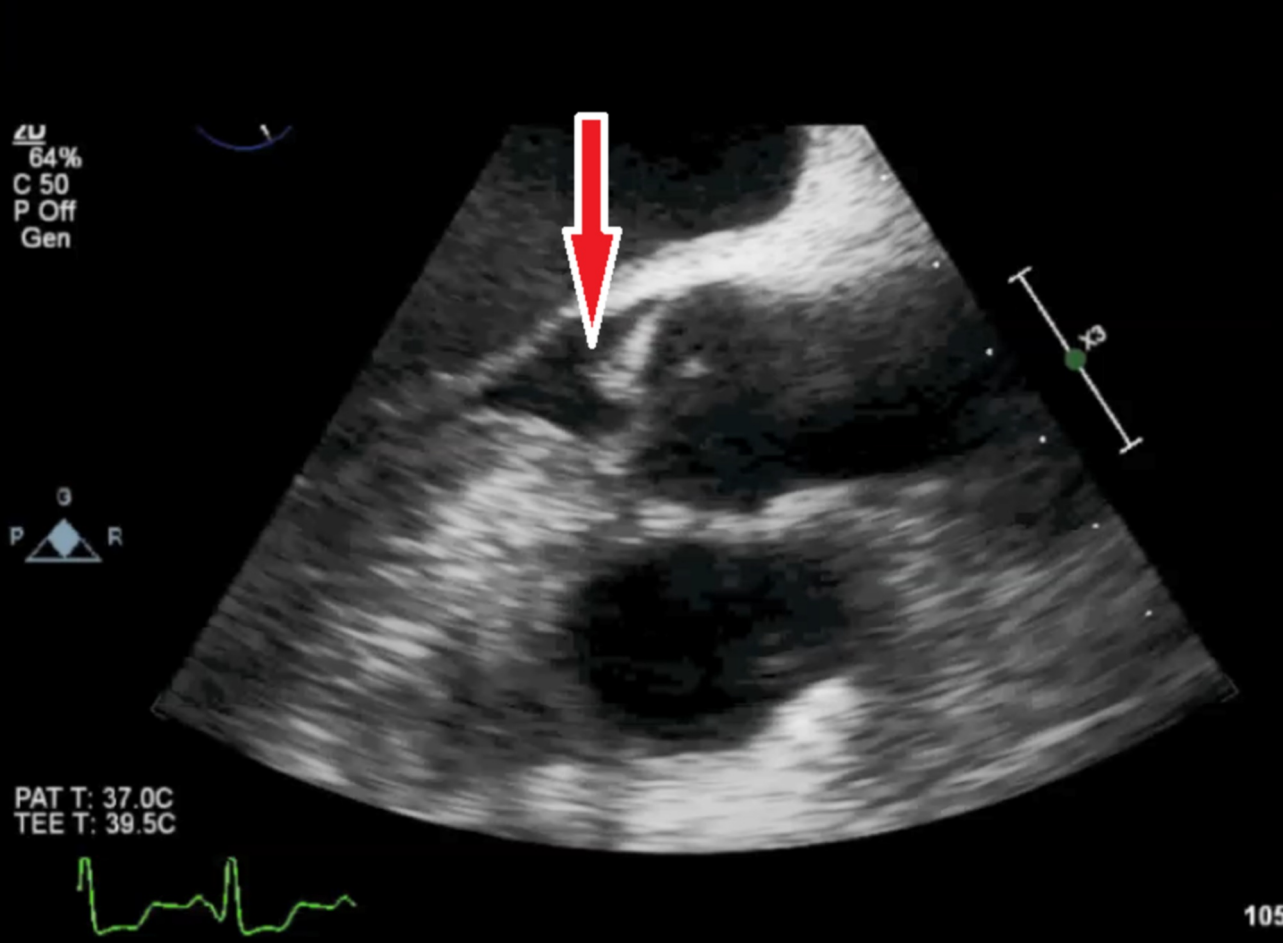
Transesophageal echocardiography (TEE) showing mass attached to the cusp of the aortic valve
